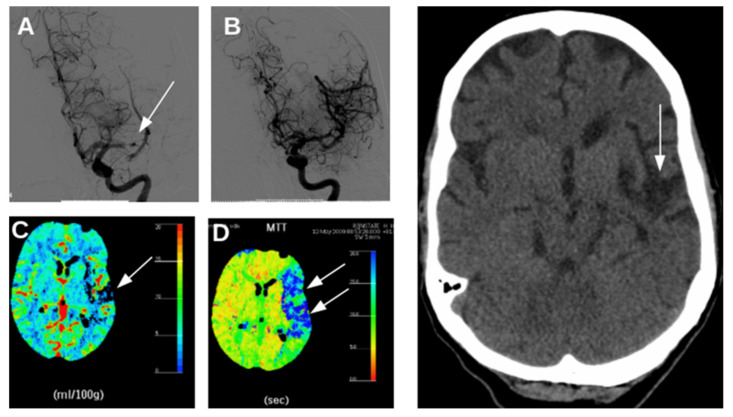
Radiology images from a patient with an acute ischemic stroke caused by occlusion of the left middle cerebral artery. (A): Digital subtraction angiography at 3 h after symptom onset obtained by intra-arterial administration of contrast agent, conforming middle cerebral artery occlusion. (B): revascularization after mechanical thrombectomy. (C): CT-perfusion maps, showing reduced cerebral blood volume (CBV) in a small part of the left hemisphere reflecting the infarct core (arrow) and (D) prolonged mean transit time (MTT) in a large part of the left hemisphere (blue tones in right panel, white arrows) reflecting hypoperfusion in the penumbra. Right: CT scan of the head 3 months after thrombectomy, showing a remaining lesion at the side of the previous infarct core (arrow), but recovery of brain areas that were part of the penumbra. The patient recovered completely.
